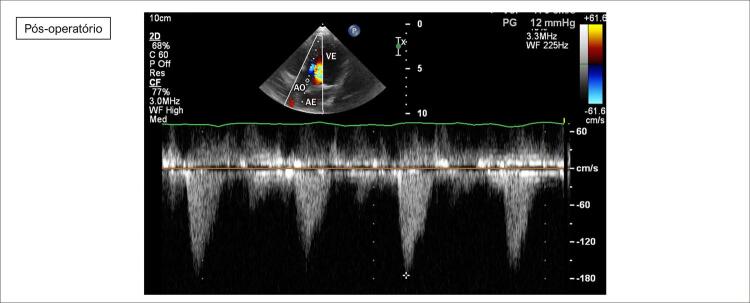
Corte apical cinco câmaras, demonstrando redução do gradiente em via de saída de ventrículo esquerdo ao color Doppler (12 mmHg) após miectomia. VE: ventrículo esquerdo; AE: átrio esquerdo; AO: aorta.
